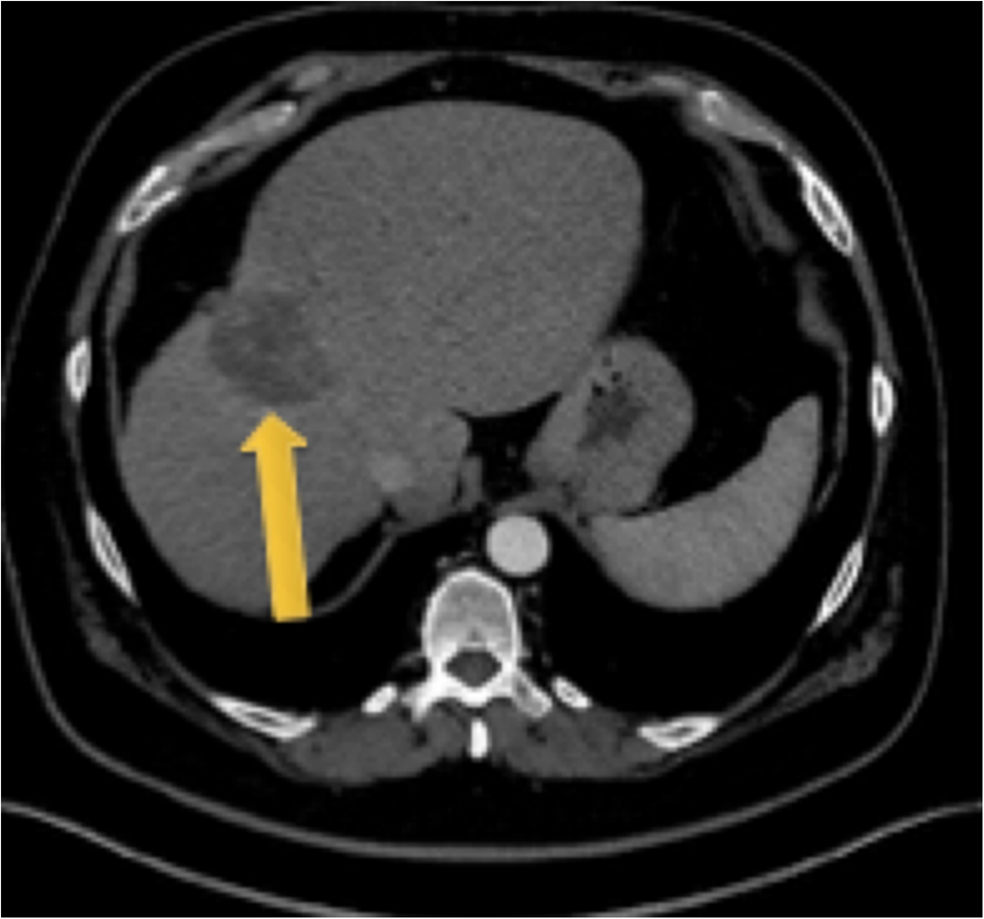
Contrast-enhanced CT following two SIRT treatments. The tumor had decreased in size to a volume of 63 cm^3^, and the FLR increased to 1469 cm^3^ rendering the patient suitable for surgical resection
